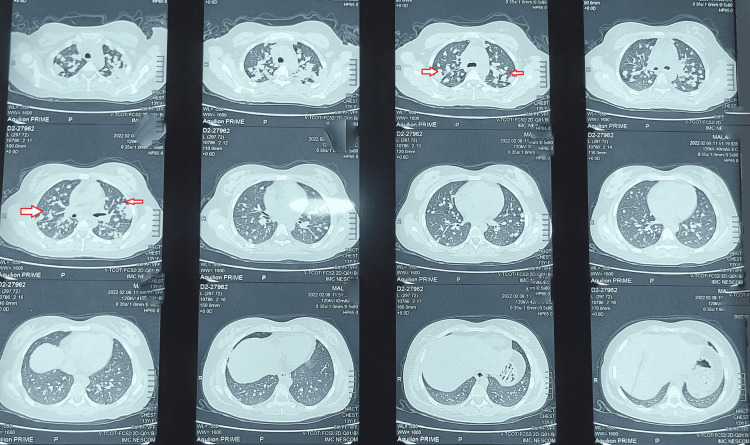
Chest CT scan showing infiltrates in both lung fields. Arrows point toward multiple diffuse infiltrates.
